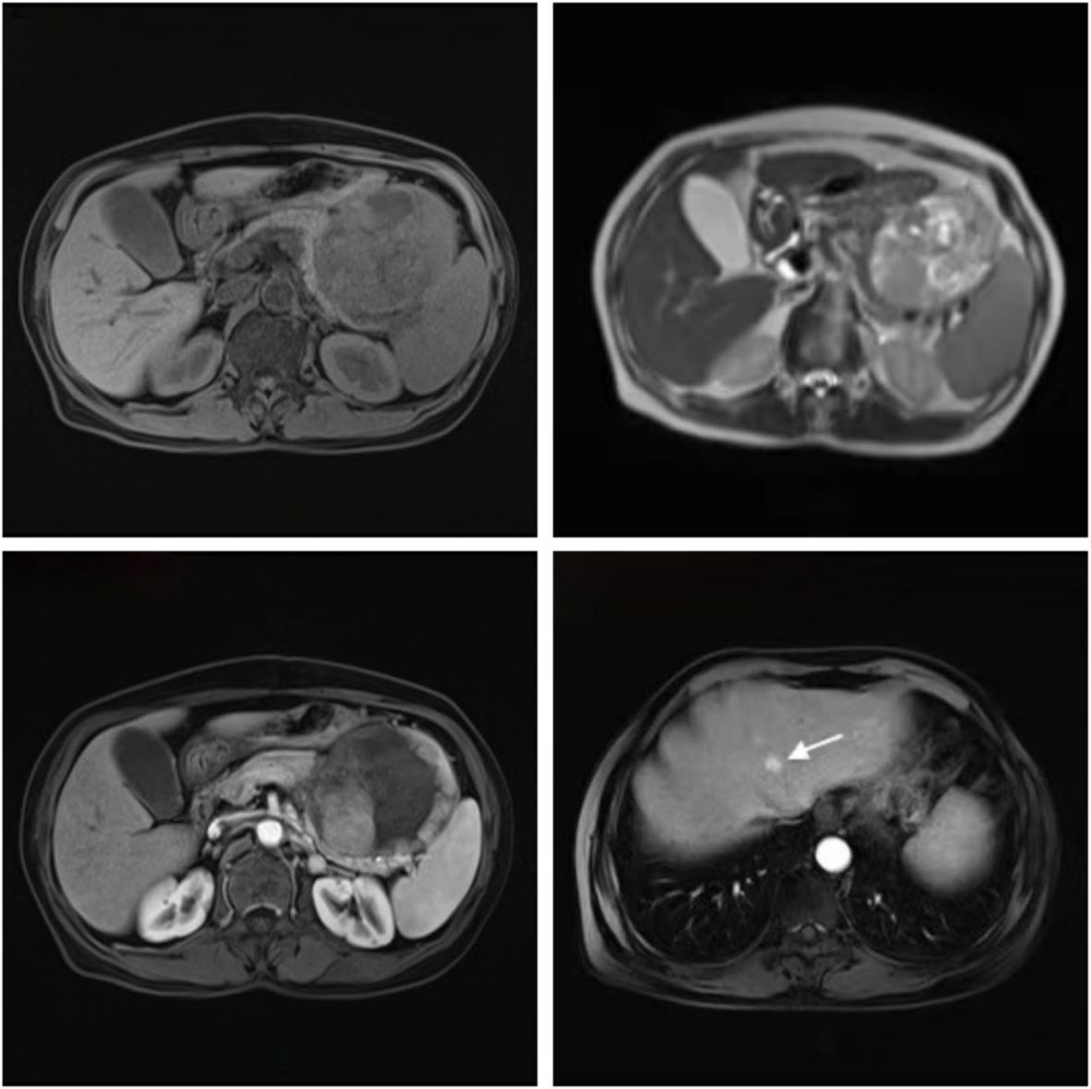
MRI image of pancreatic FDCS. a MRI scan showing a mass at the tail of the pancreas, with T1WI mixed signal mainly isosignal. b T2WI showing slightly higher signal. c Enhanced scan showing uneven and significant strengthening and the cystic region remaining unenhanced. d Multiple persistent strengthening nodules in the liver (white arrows)
